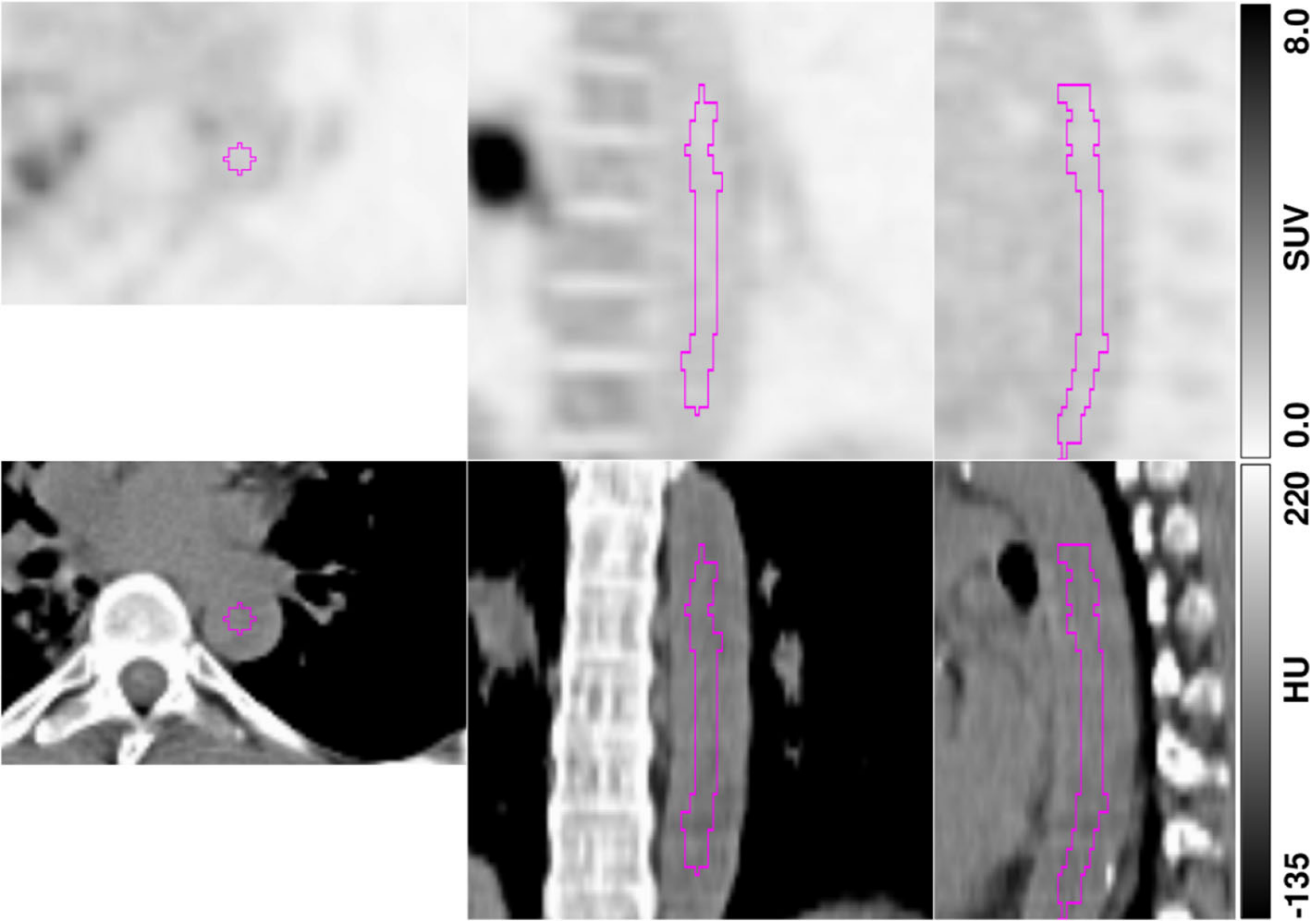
Example of aorta ROI delineation (top, PET; bottom, CT). Delineation was performed in the transaxial CT slices and transferred to the PET image volume. Shown are orthogonal slices through the 3D-ROI (magenta line)
